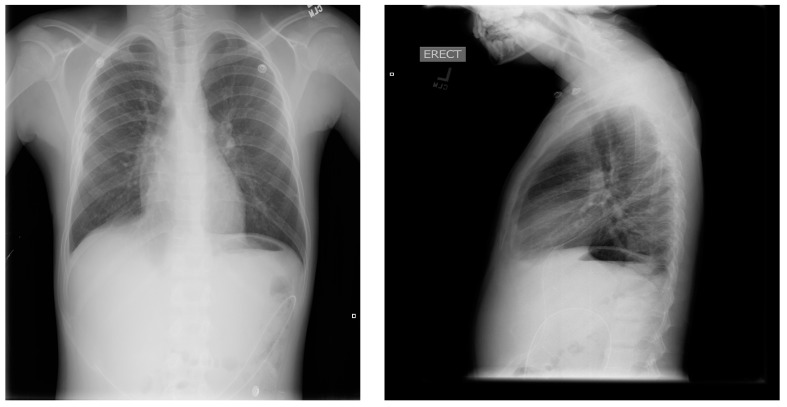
Post-operative chest X-rays, showing reversed pleuroperitoneal shunt in right chest and abdomen.
